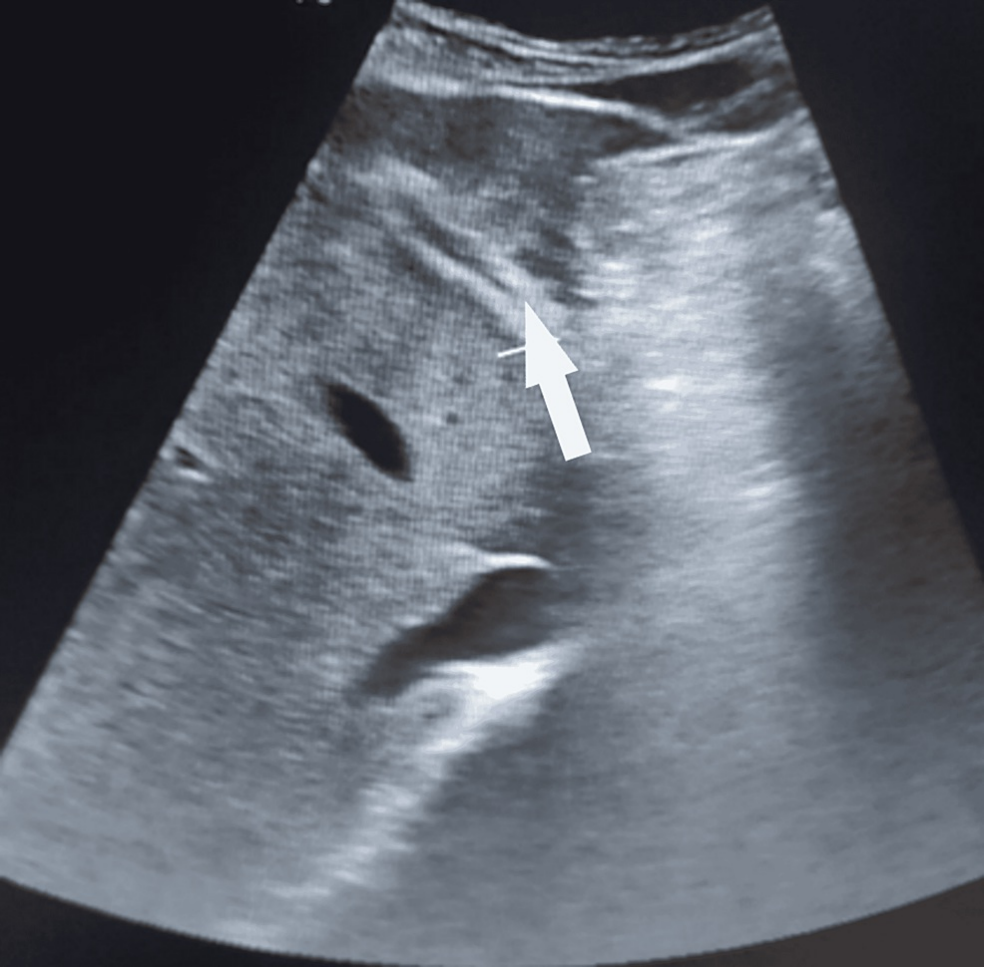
Abdominal ultrasound demonstrating thickening and intrahepatic bile ducts (white arrows)
